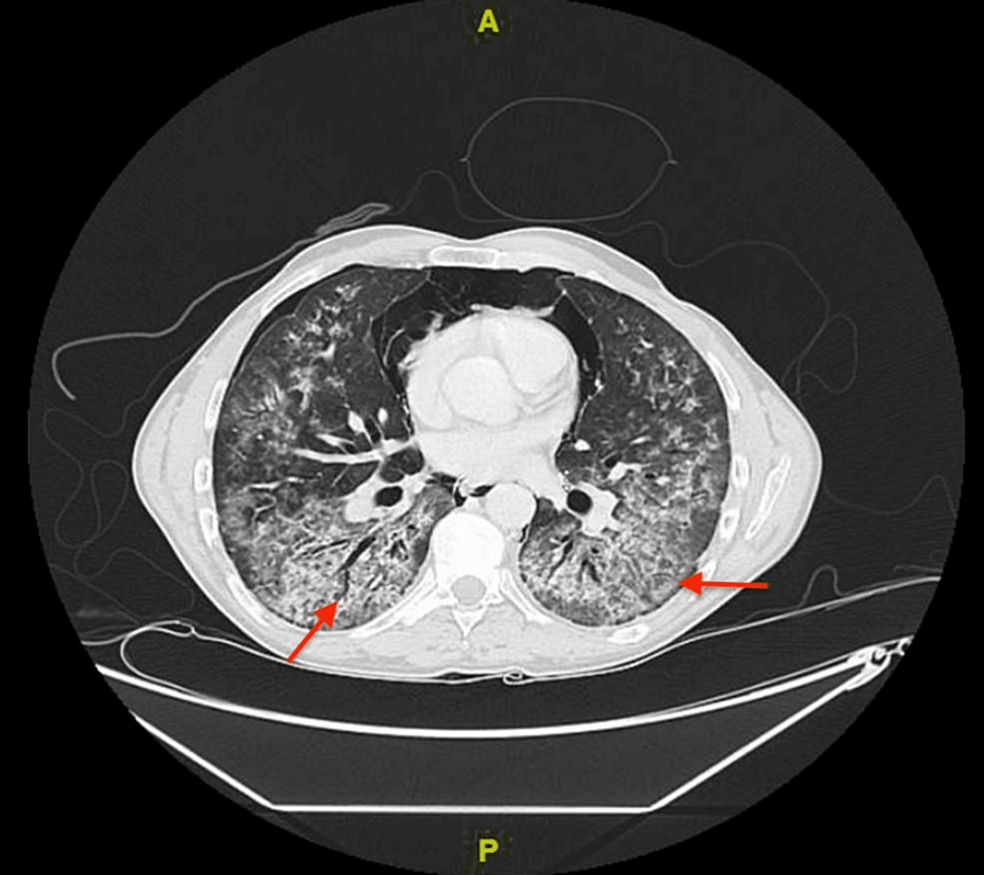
Chest CT scan Chest CT with contrast showed patchy bilateral dependent ground glass opacities with associated tree-in-bud nodules along with interlobular septal thickening and traction bronchiectasis suggestive of lung inflammation on top of interstitial lung disease.
